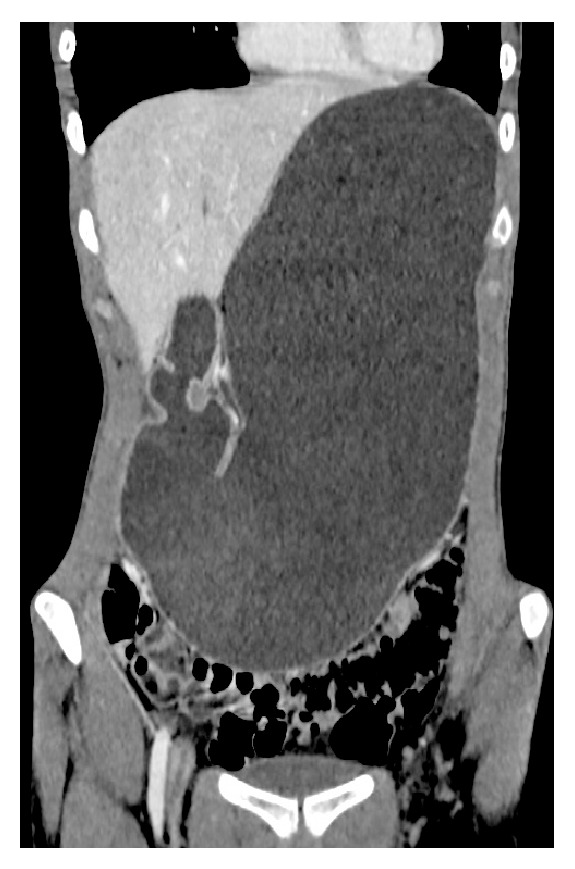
Coronal CT section demonstrating gross distension of the stomach.
